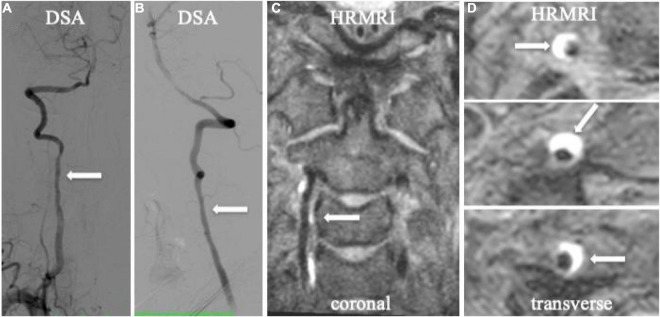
Spiral intramural hematoma in the patient with vertebral artery dissection. Digital subtraction angiography (DSA) showed irregularly mild stenosis without any dissecting signs on anteroposterior and lateral projections (A,B, arrow). T1-weighted HRMRI showed an spiral intramural hematoma in the right vertebral artery in the coronal (C, arrow) and transverse planes (D, arrow).
